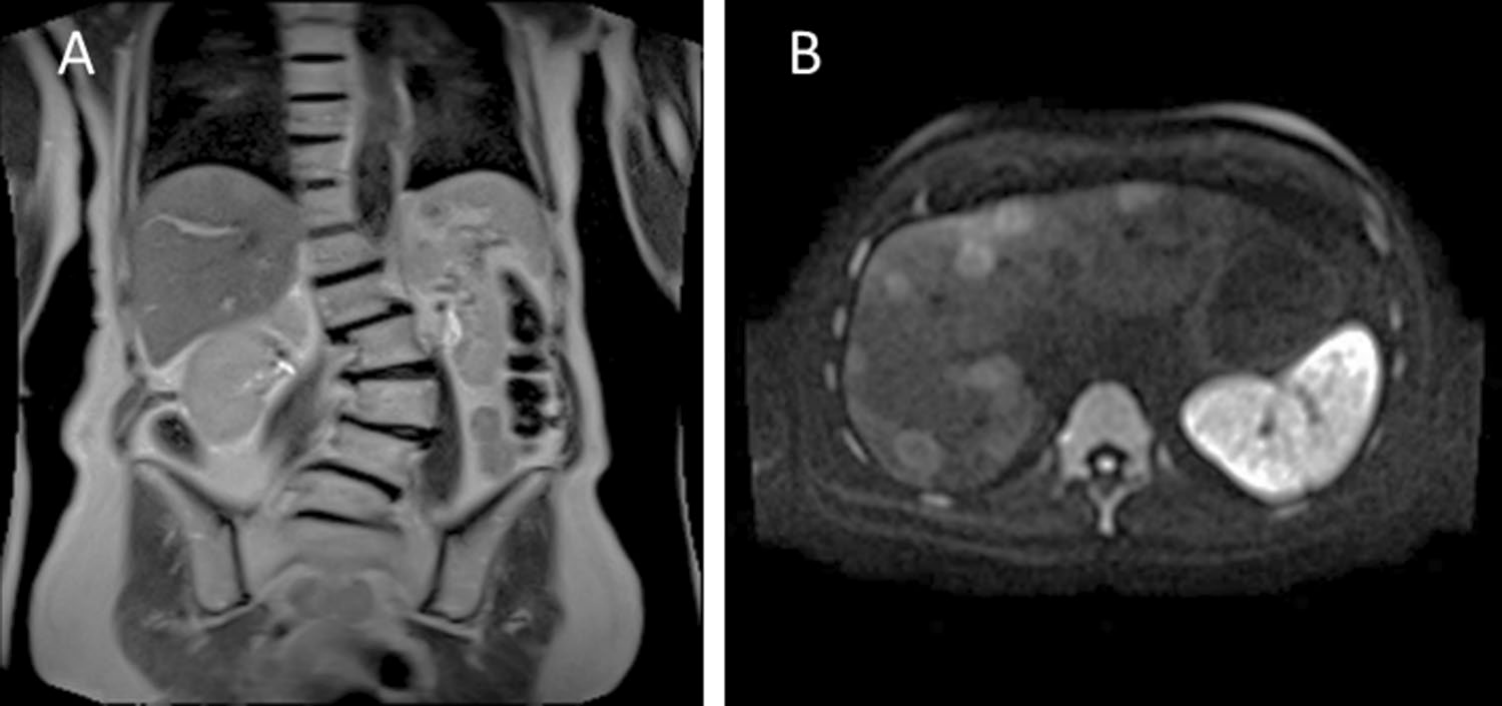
A Coronal T2 HASTE image of the abdomen and B DWI-EPI of the liver acquired at 0.55 T (FreeMax, Siemens; Erlangen, Germany). Imaging parameters: T2 HASTE: PAT 3, TR = 1000 ms, TE = 92 ms, 1.5 × 1.5 × 6.0mm^3^, TA: 3:07 min. DWI EPI: PAT 2, 1.6 × 1.6 × 6.0 mm^3^, 4:26 min, b-factor: 800 (courtesy University Hospital Erlangen)
